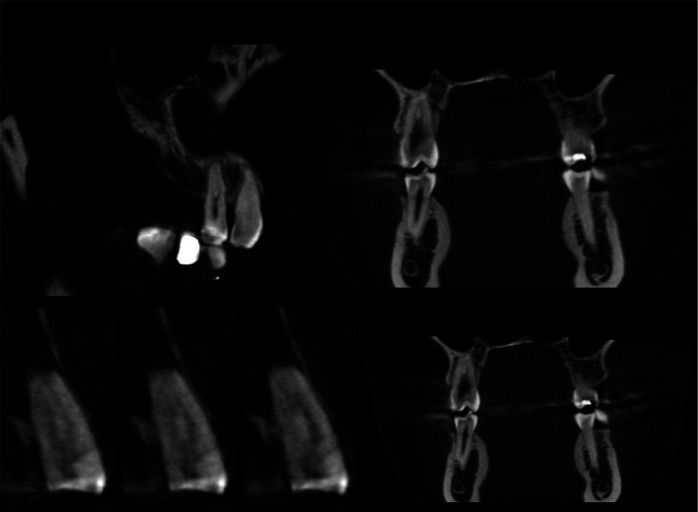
The coronal and sagittal CBCT (Cone-Beam Computed Tomography) view of secondary maxillary premolar with single root
